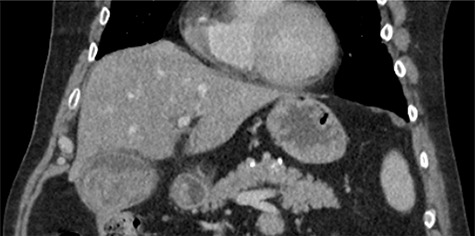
Coronal non-contrast CT of abdomen on day of presentation, showing hyperdense nodular soft tissue filling the gallbladder lumen.
